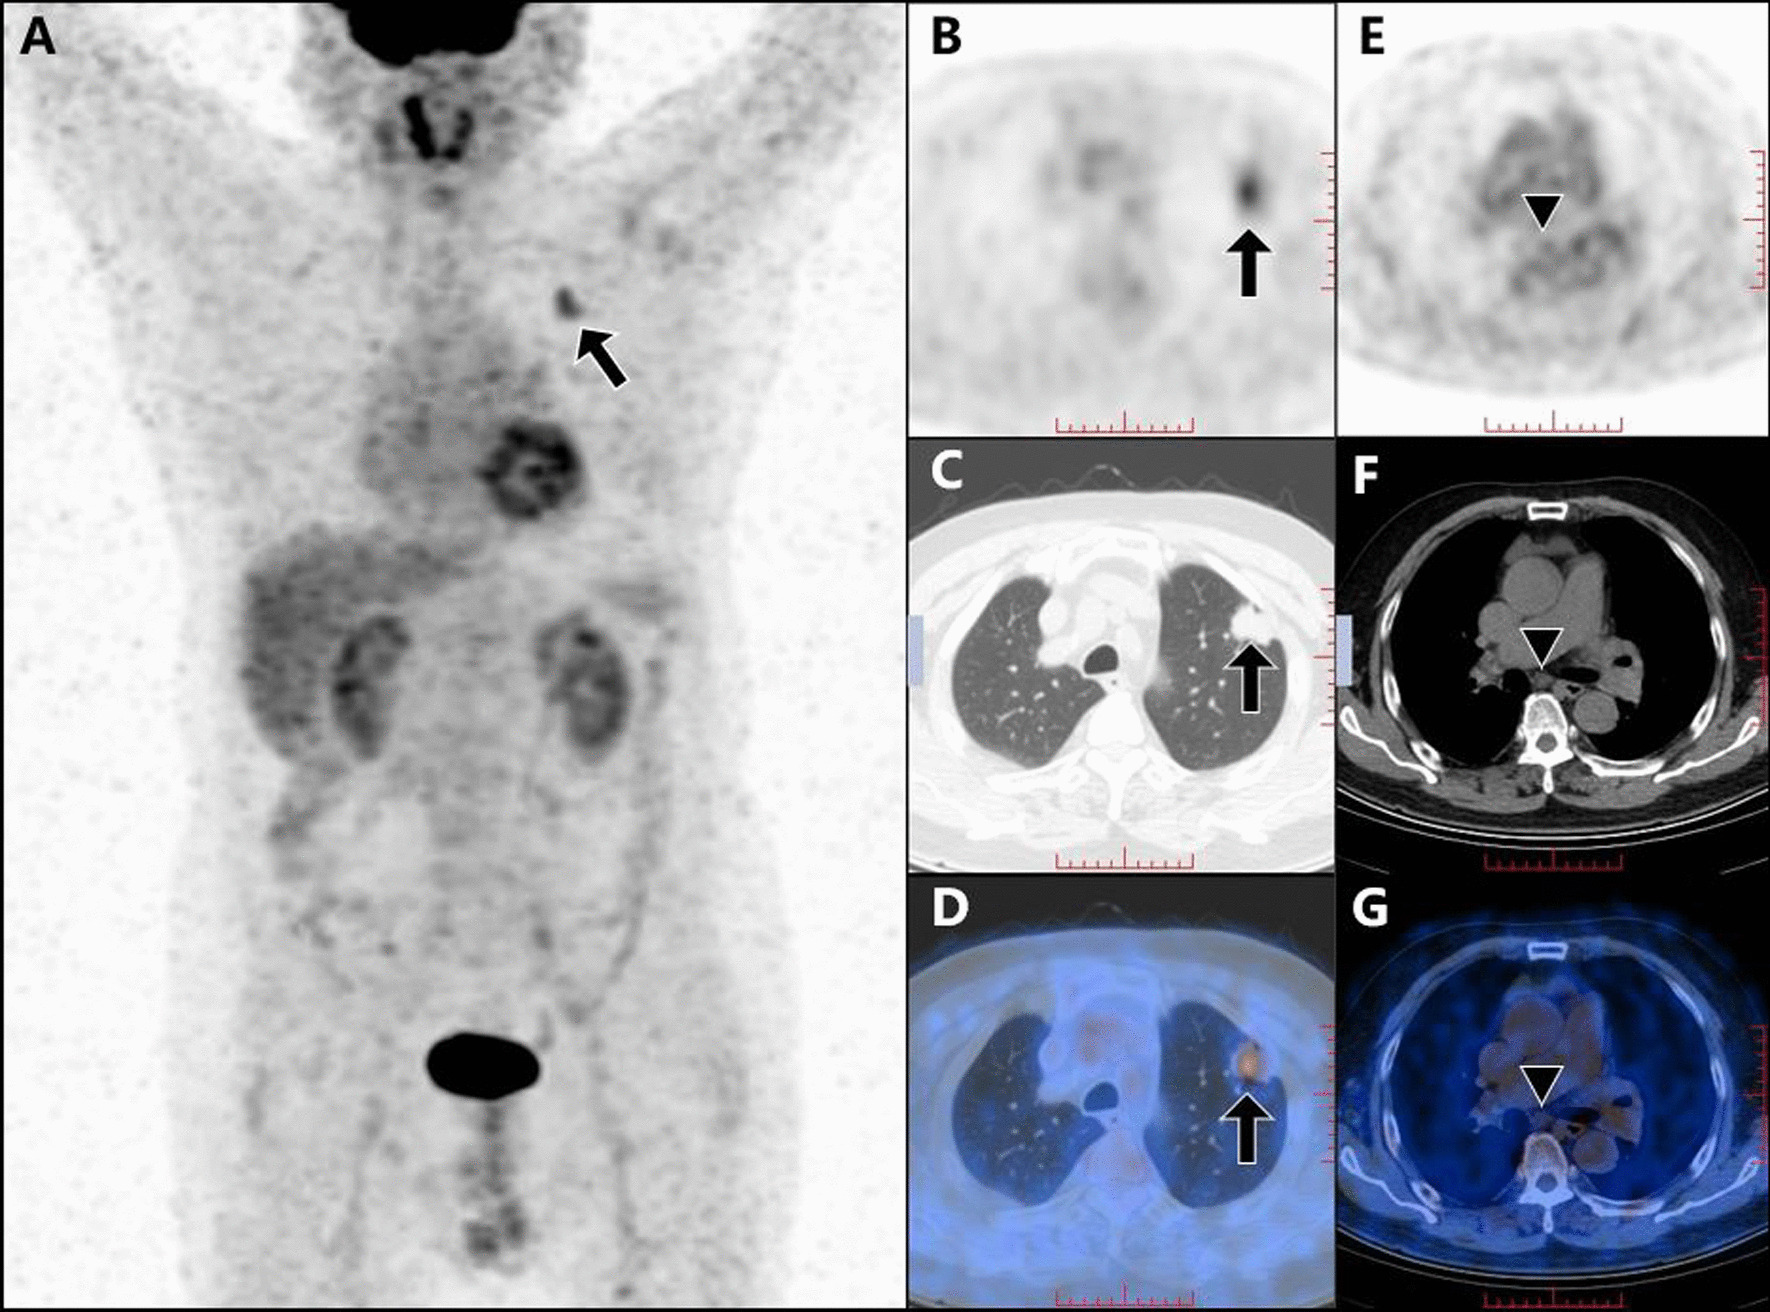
An elderly male (A–G) with low-level SUVmax (2.04) of mediastinal lymph node, low-level primary-tumor SUVpeak (3.34), low serum CEA (1.69 ng/ml) and high serum SCC (2.5 ng/ml). The primary tumor, which was confirmed as lung adenocarcinoma through operative pathological assay, is shown in ^18^F-FDG PET/CT maximum intensity projection (MIP) image (A), PET (B), CT (C) and PET/CT (D) images (arrows). The mediastinal lymph node in area 7, which was the highest-SUVmax (2.04) node in the mediastinum and proved to be negative by postoperative pathological analysis, was displayed in ^18^F-FDG PET (E), CT (F) and PET/CT (G) images (triangles)
